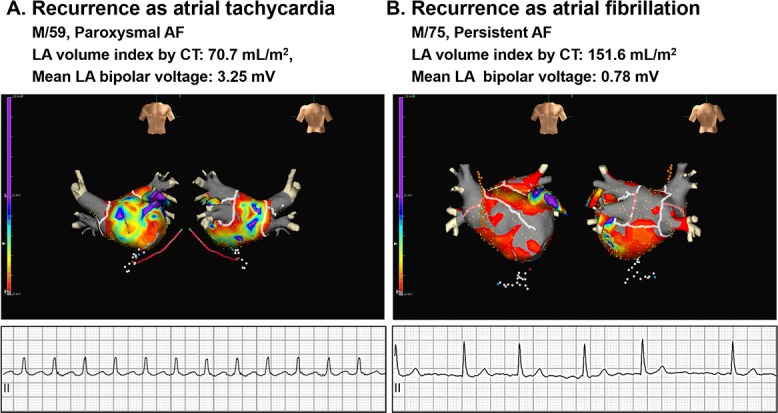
Typical examples of patients with recurrent AT and recurrent AF. Patients with recurrent AT (A) have a relatively smaller LA volume index and higher mean LA bipolar voltage than patients with recurrent AF (B). AT = atrial tachycardia; AF = atrial fibrillation; CT = computed tomography; LA = left atrial.
